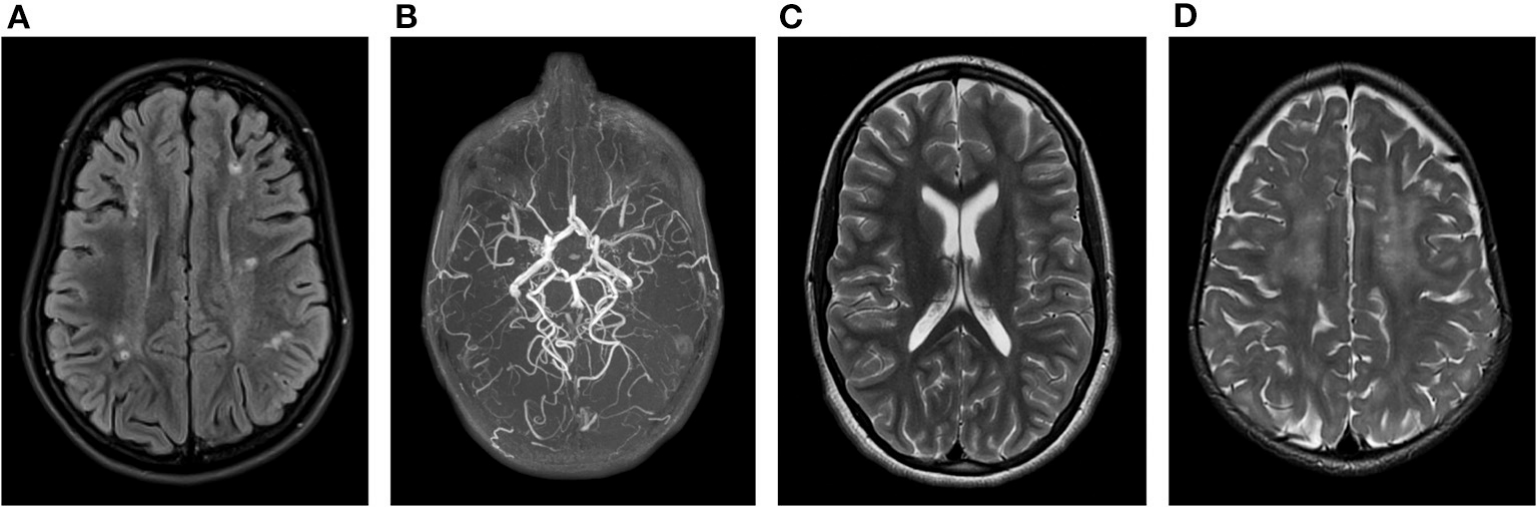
Brain MRI findings in our AGS patients: (A) Periventricular and frontoparietal lesions suggestive of cortical and white matter infarctions associated with (B) moyamoya disease at the distal left internal carotid artery and the proximal right middle cerebral artery on MR angiography in patient 1. (C) Isolated posterior thinning of the corpus callosum in patient 2. (D) Bilateral frontal periventricular microcysts and diffuse white matter hyperintensities in patient 3.
